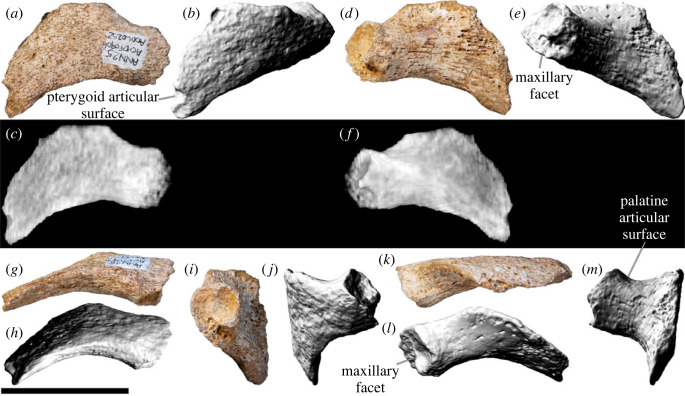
Diamantinasaurus matildae* referred left ectopterygoid (AODF 0906). (*a–m*) Left ectopterygoid in (*a–c*) anterior, (*d–f*) posterior, (*g*,*h*) ventral, (*i*,*j*) lateral, (*k*,*l*) dorsal and (*m*) posterior views. (*a*), (*d*), (*g*), (*i*) and (*k*) are photographs; (*b*), (*e*), (*h*), (*j*) and (*l–m*) are three-dimensional models derived from surface scans; and (*c*) and (*f*) are three-dimensional models derived from CT scans. Scale bar = 50 mm.
